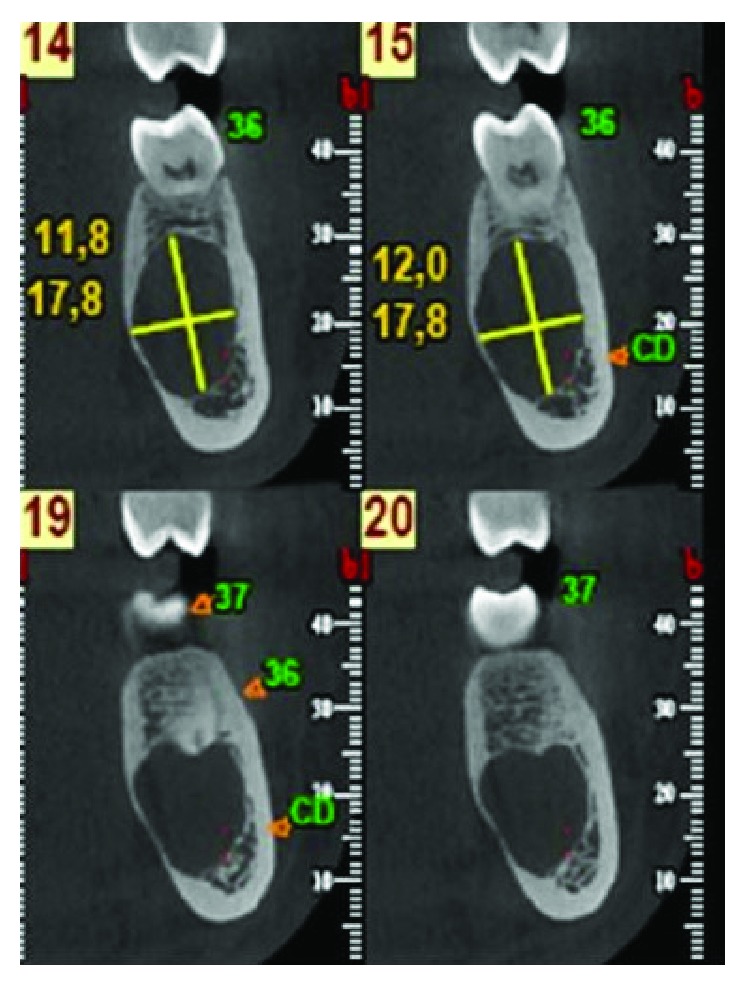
Cone beam computerized tomography. A well­defined unilocular radiolucent lesion of 12.0 × 17.8 mm is observed, with preservation of both the root apex and the cortical bone. However, a marked thinning of the lingual cortical mandibular plate is seen.
